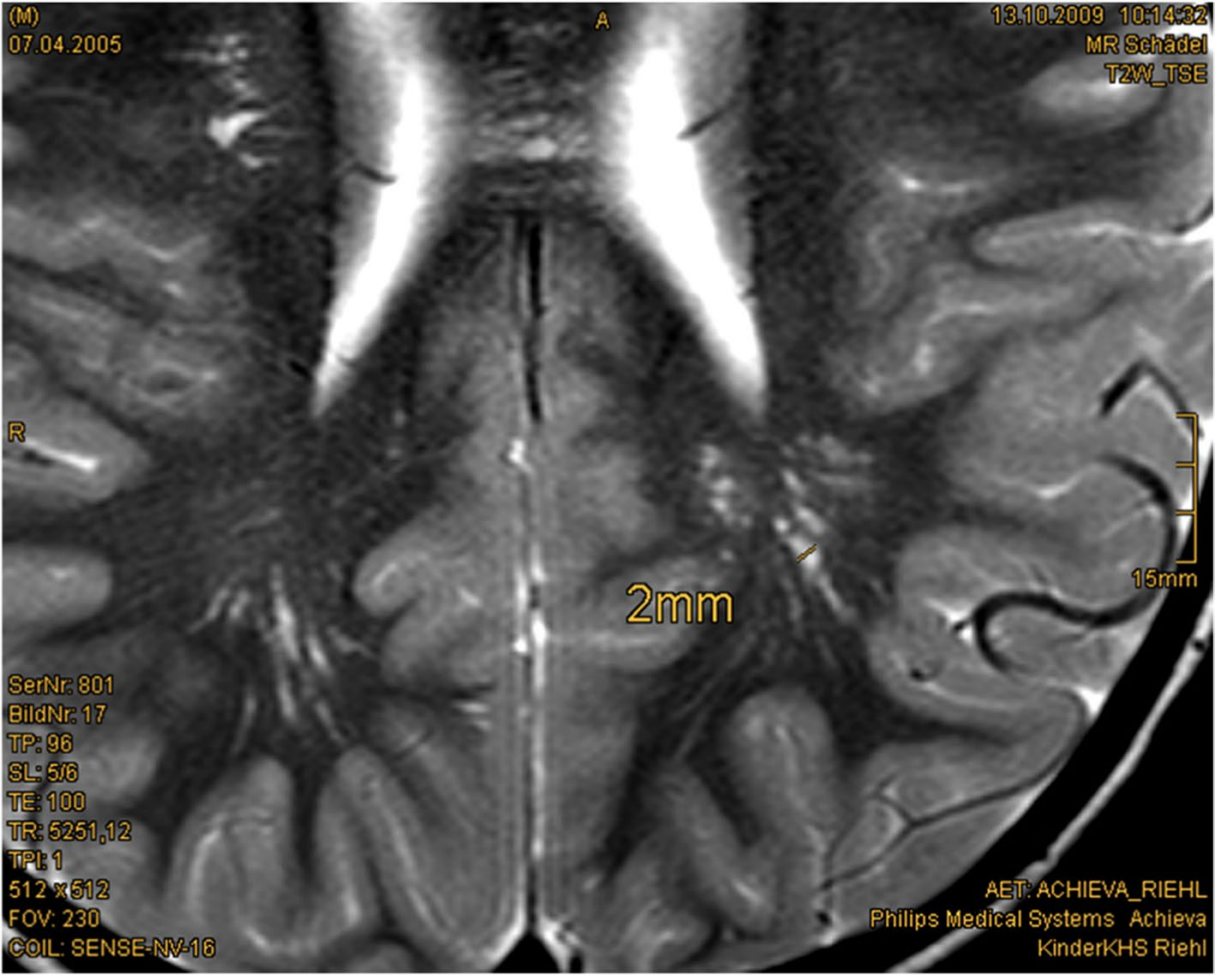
Enlarged perivascular spaced (EPVS) in cerebral MRI: 4.5-year-old boy, T2w-image, EPVS 2 mm diameter [Courtesy Kinderkrankenhaus Kliniken der Stadt Köln]
